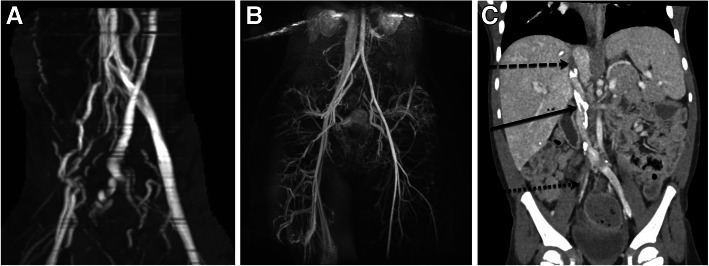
a MRV of an 18 y/o M with chronic right lower extremity swelling and DVT demonstrates numerous venous collaterals but no central draining vein beyond the level of the iliac veins. This delayed, post-contrast MIP image shows the numerous venous collaterals in the pelvis and along the spine. b MRV of a 16 y/o F with acute onset of left lower extremity DVT shows patency of the right iliac veins draining into the IVC but no opacification of the left iliac veins. The overriding right common iliac artery compresses the left common iliac vein leading to the delayed venous drainage of the extremity and eventual thrombus formation. c CT of a 4 y/o M with chronic occlusion of the IVC (dashed arrow), multifocal calcified thrombi in the IVC (solid arrow), and fresh acute clot in the right common iliac vein (dotted arrow). A CT exam in this child allowed for an accurate, high resolution exam for deep venous recanalization planning without having to put the child under general anesthesia to obtain the images
